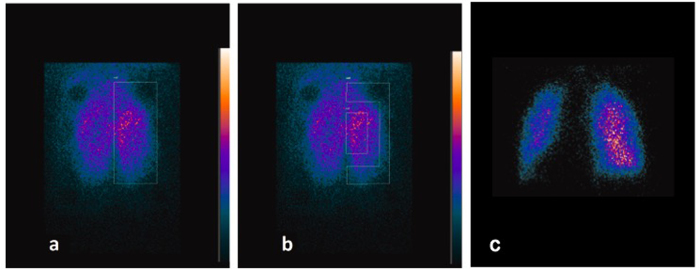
81mKrypton planar scintigraphic images with the regions of interest (ROIs). (a) Left ROI. (b) Central (20% of the lung) and peripheral (50% of the lung) ROIs. (c) A human planar scintigraphy of normal lungs from the evaluation of a patient in the ward leading to the conclusion of a normal lung function.
